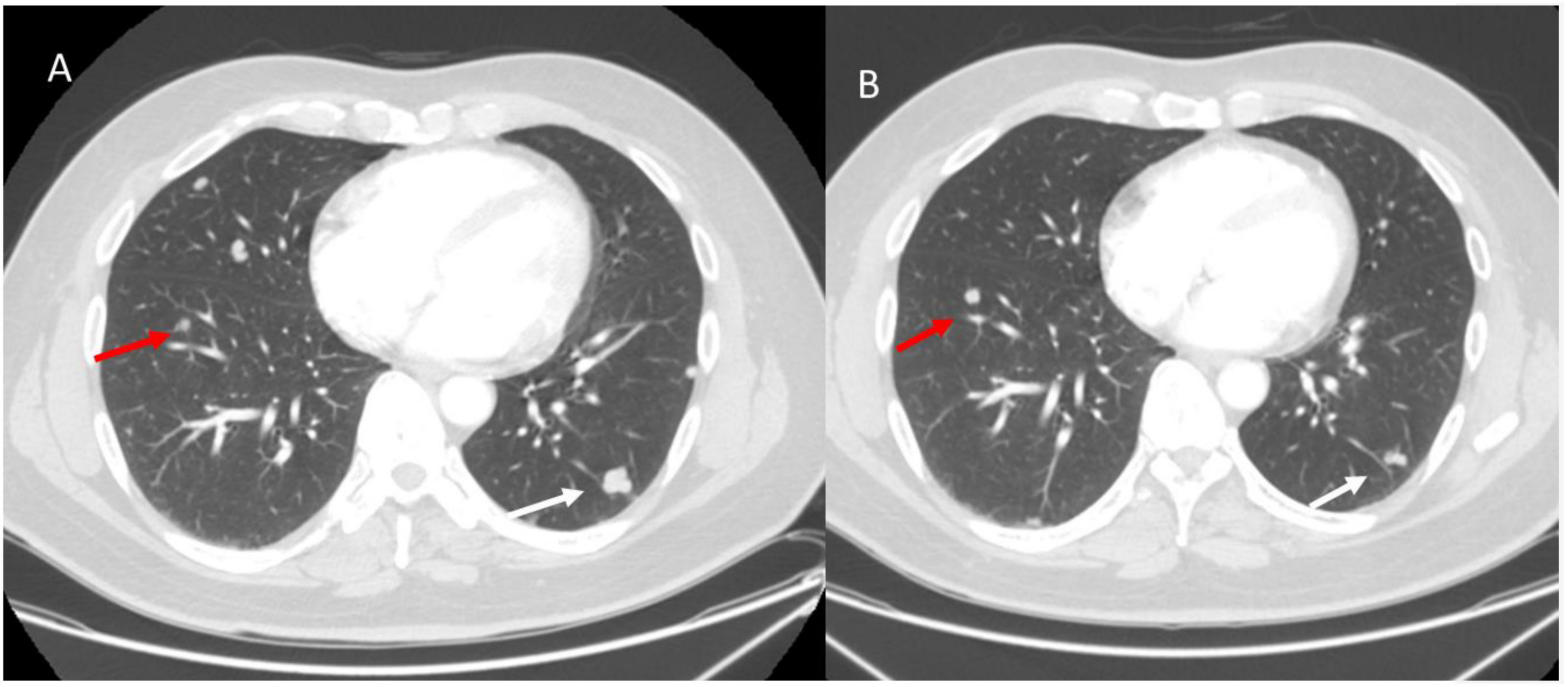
Dissociative response in a melanoma patient with lung metastases undergoing immunotherapy. Axial CT scans: (A) (Baseline CT, Pre-Treatment): Two metastatic lung lesions are identified: one in the left upper lobe (red arrow) and another in the right lower lobe (white arrow). Both lesions appear well-circumscribed with peripheral subpleural distribution. (B) (Follow-up CT After 3 Months of Immunotherapy): The right lower lobe lesion (white arrow) shows a significant reduction in size, indicating a partial response to immunotherapy. However, the left upper lobe lesion (red arrow) has increased in size, suggesting progressive disease in this specific lesion despite the overall tumor burden decrease. From Vanzulli and Albano, (42).
